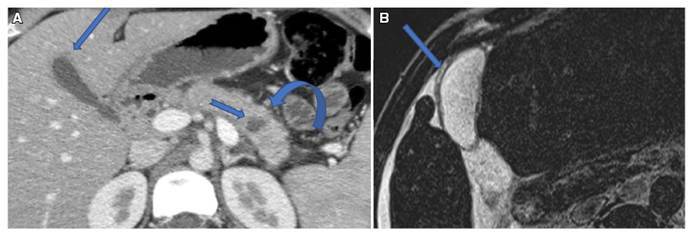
AIP (A) CT scan showing GB wall thickening (arrow), cystic pancreatic duct dilatation (Notched arrow), Halo sign (curved arrow) (B): AIP with associated circumferential gall bladder wall thickening. (Arrow)
